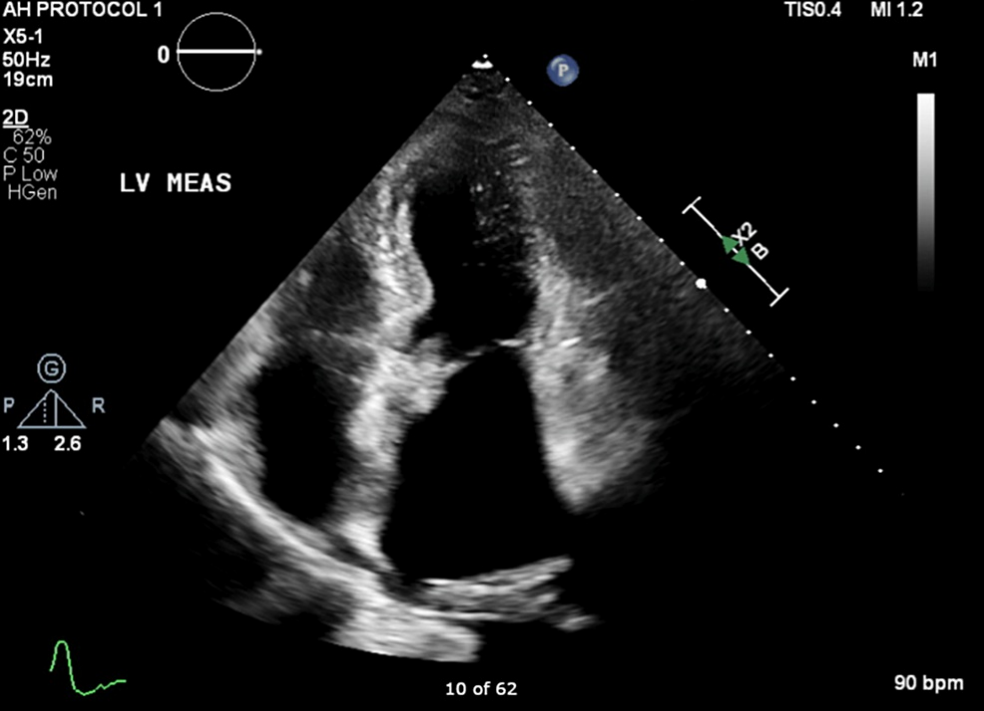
Repeat transthoracic echo without the left atrial mass
